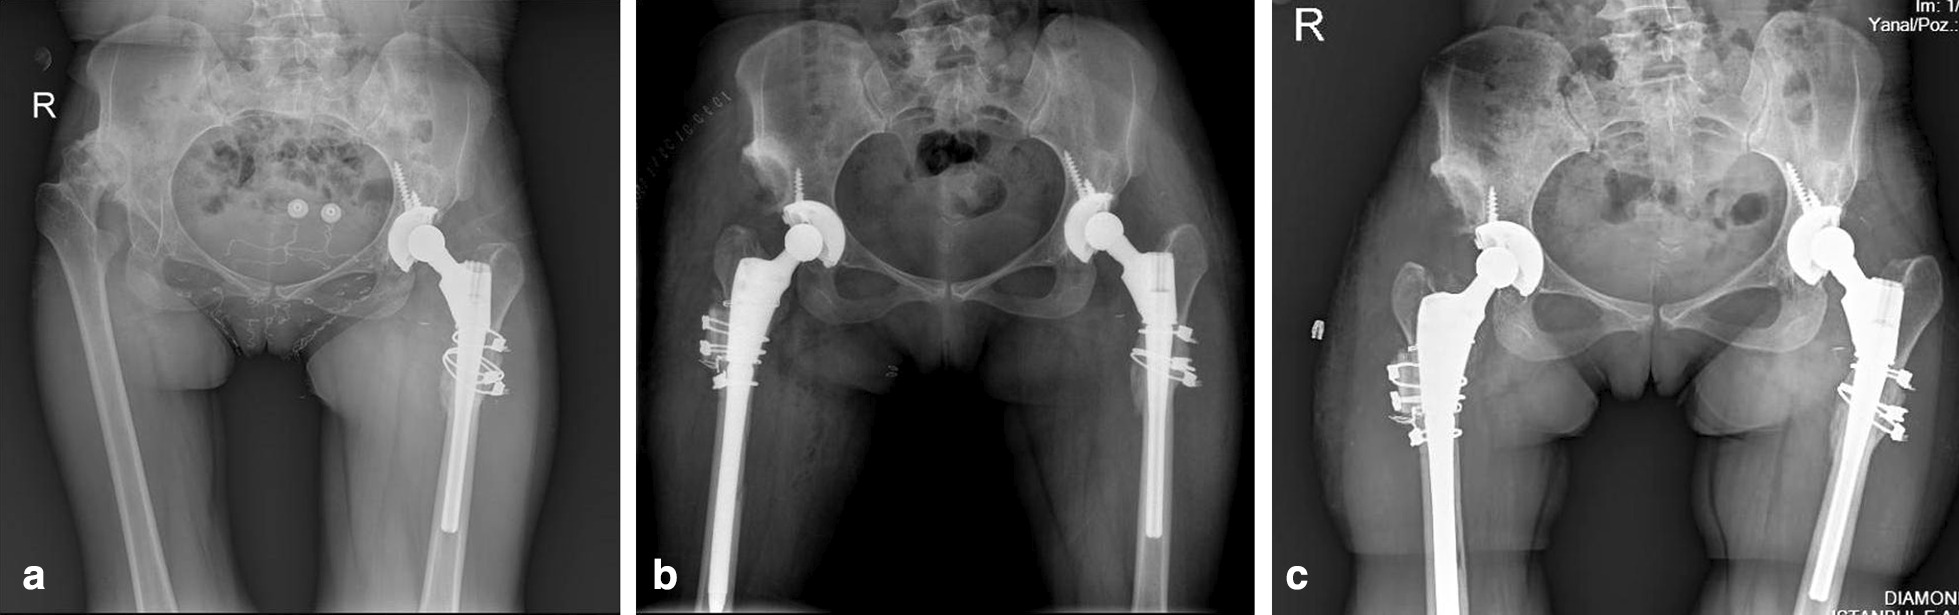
Radiographs of a 62 years old female with high hip dislocation. a Preoperative anteroposterior view. b Early postoperative radiographic image. Asetabuler componenet was reconstructed in the true acetabulum with transverse osteotomy. c Postoperative radiographic image at 5 years follow-up, bone union was detected at the osteotomy site
